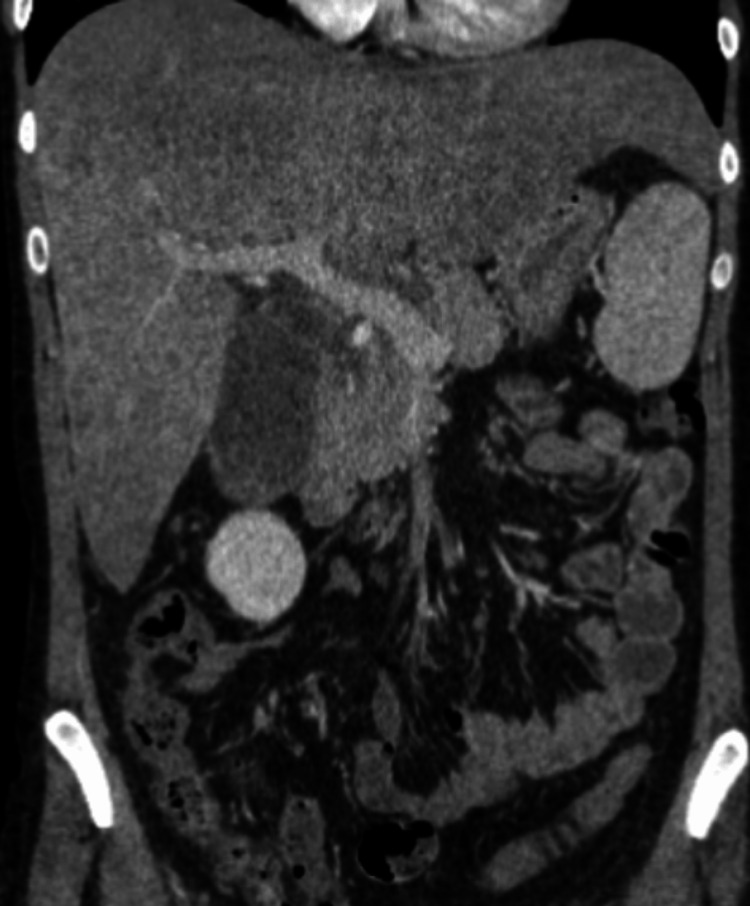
Coronal view of computed tomography (CT) of abdomen showing massive hepatomegaly
